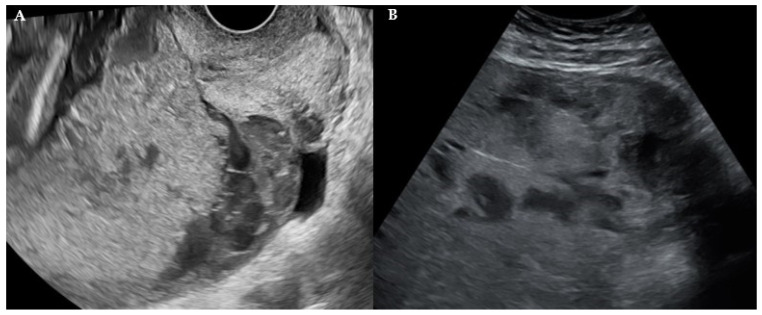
Representative ultrasound findings suggestive of placenta accreta spectrum in women with placenta previa. (A) Transvaginal grayscale ultrasound demonstrating loss of the retroplacental clear zone with multiple irregular intraplacental lacunae. (B) Transabdominal grayscale ultrasound demonstrating absence of the retroplacental clear space with heterogeneous placental echotexture at the lower uterine segment.
